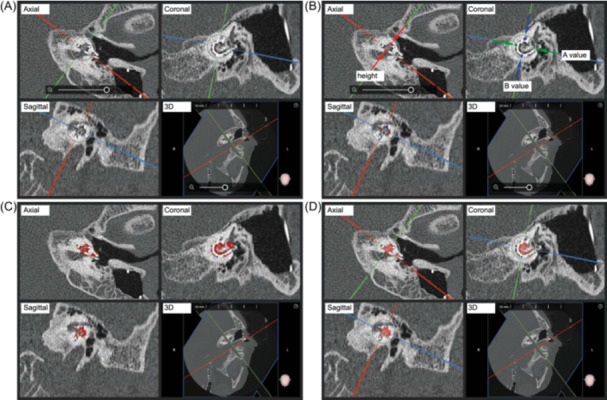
Measurement of cochlear duct length. (A) Axial computer tomography (CT) image of the temporal bone. (B) In the coronal view, the *A*‐value (green arrows) and the *B*‐value (blue arrows) are shown. (C) The red overlay indicates the automated detection of the electrode array. (D) Visualization of the software's automated identification.
